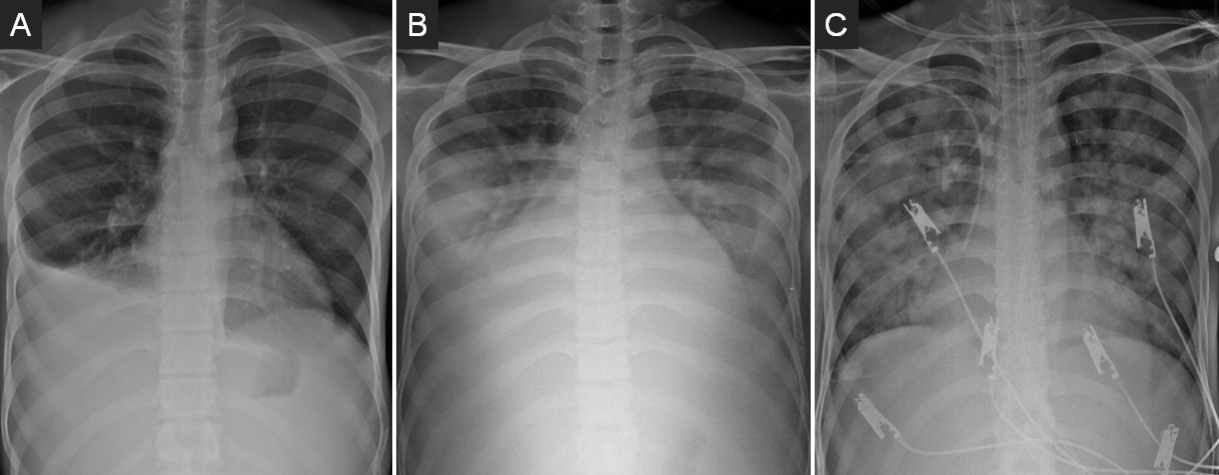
Chest radiographies presenting (A) right basal consolidation, pleural effusion (admission), (B) bilateral pleural effusion (day 21), and (C) extensive bilateral interstitial infiltrate with diffuse areas of consolidation (day 26).
